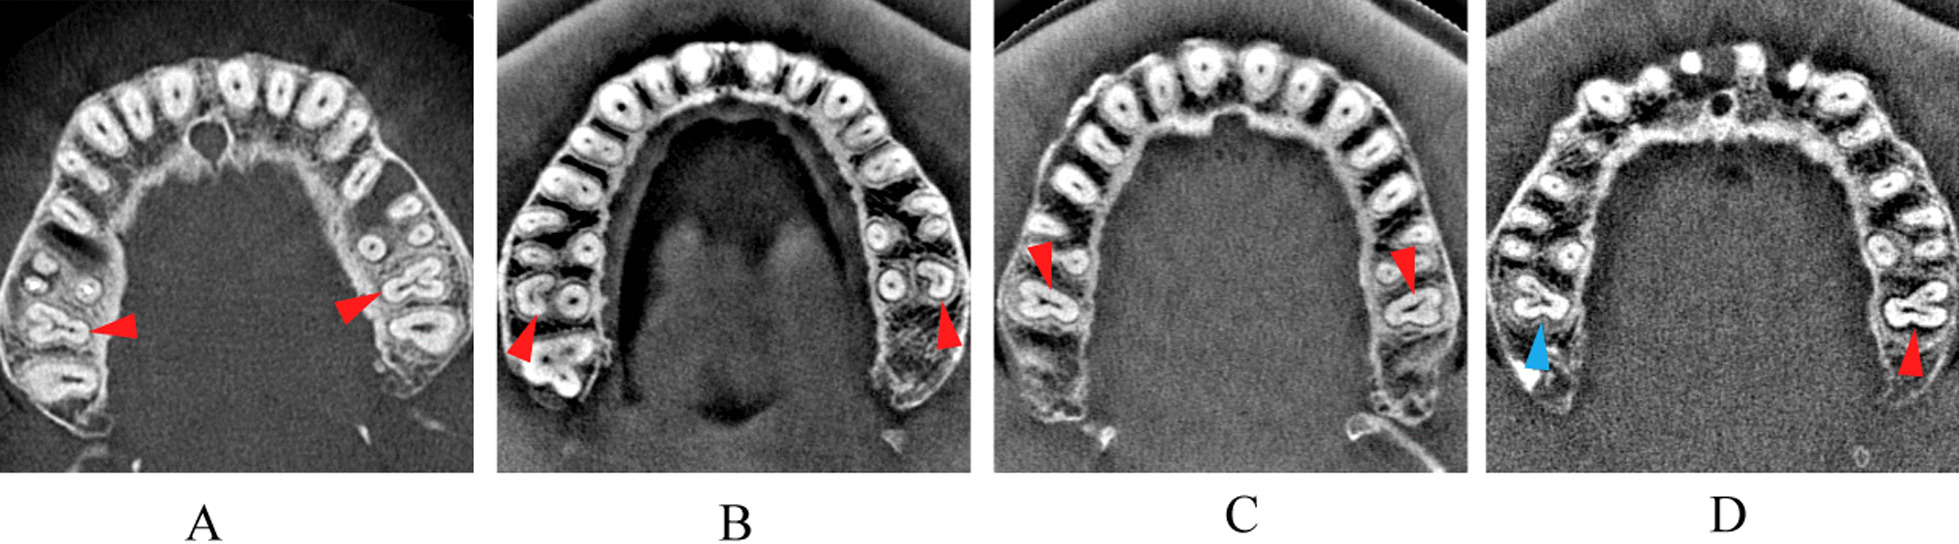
Represent CBCT images of bilateral presence of C-shaped canals in MSMs and MSMs. A, red arrow showed biliteral presence of Type II (MB-P-DB) C-shaped root canals. B, red arrow showed biliteral presence of type I subtype C (MB-DB) C-shaped root canals. C, red arrow showed biliteral presence of type I subtype A (MB-P) C-shaped root canals. D, red arrow showed the presence of Type I subtype B (DB-P) C-shaped root canals, and blue arrow showed the presence of Type II (MB-P-DB, V shape) C-shaped root canals
